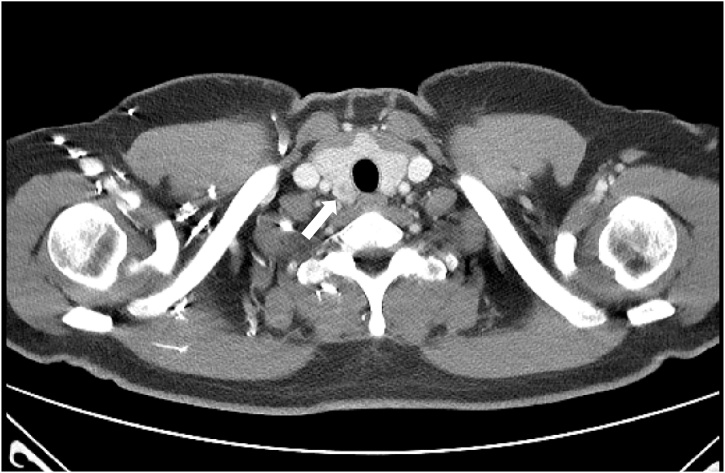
Chest computed tomography scan: a hypodense nodule in the right lobe of thyroid gland (white arrow).
